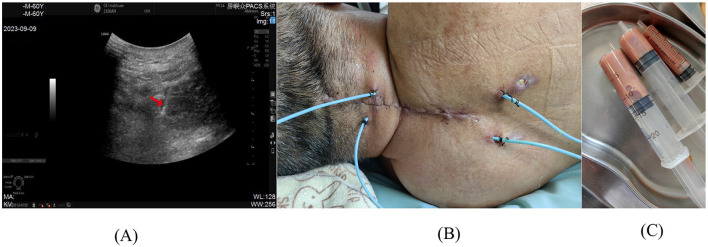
(A) Ultrasonographic needle trajectory during catheter placement, with arrow indicating the puncture needle; (B) External segment of the drainage catheter post-deployment; (C) Coffee-colored purulent fluid aspirated through the catheter following successful placement.
